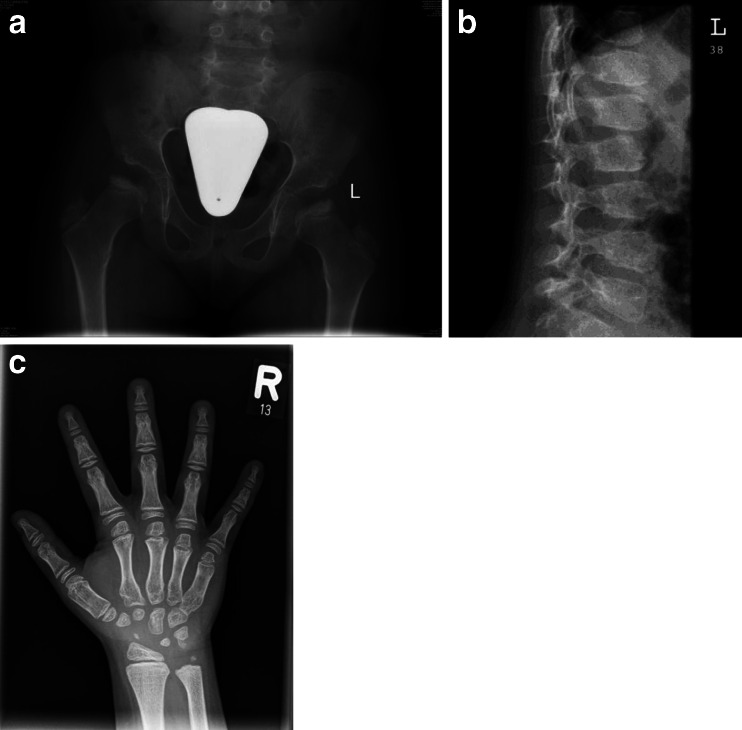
Radiographs of patient 2, female, MPS IVA. a AP pelvis and hips, age 7 years: normal pelvis; bilateral dense/dysplastic CFE. b Lateral lumbar spine, age 6 years: slightly abnormal with mild residua of the middle beaking of several vertebrae (T11, T12) and a slightly superior notched L1. c AP right hand and wrist, age 8 years: mild generalized brachydactyly; wide growth plates and hypoplastic carpal centers; no significant proximal pointing, but poor proximal metacarpal formation for age
